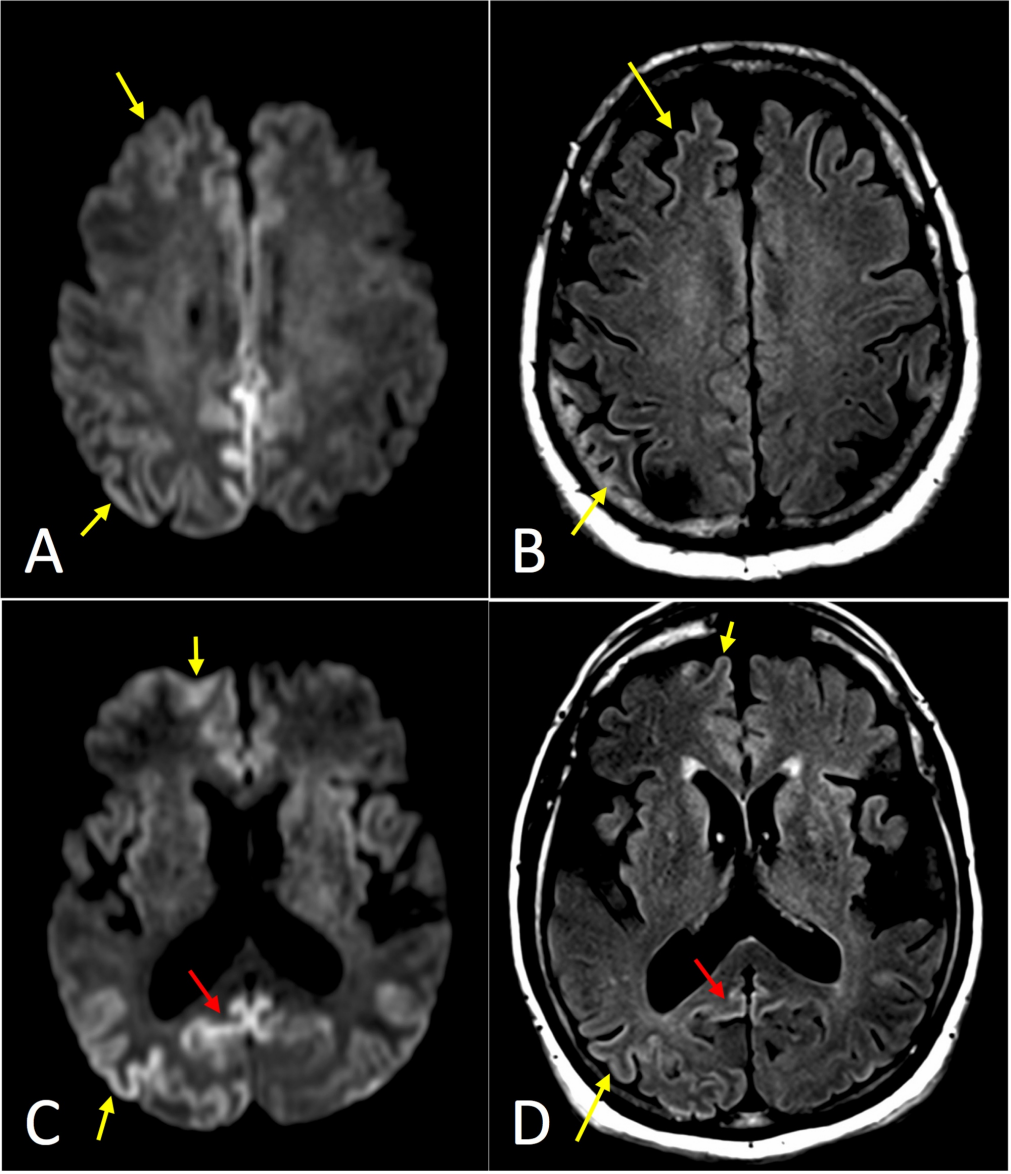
Axial magnetic resonance imaging (MRI) of Case 3. (A) Diffusion-weighted image (DWI) at the level of the centrum semiovale demonstrating asymmetrically increased signal in the frontal and parietal cortex (yellow arrows), with notable involvement of the cingulate gyrus. (B) T2-weighted fluid attenuation inversion recovery (T2-FLAIR) image with less corresponding hyperintense signal. (C) DWI at the level of the basal ganglia demonstrating asymmetrically increased signal in the neocortex (yellow arrows) and striatum, with notable involvement of precuneus (red arrow). (D) T2-FLAIR image with less corresponding hyperintense signal.
